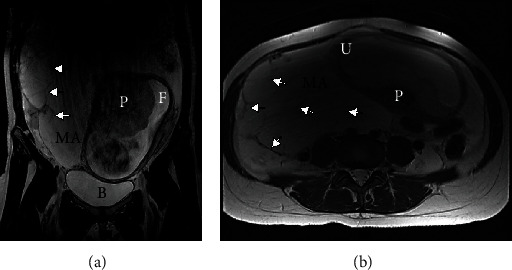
(a), (b) Abdominal and pelvic MRI without IV contrast ((a) coronal plane, (b) axial plane) shows a gravid uterus and significant amount of T2 hyperintense fluid, some of which is loculated with internal septations (arrows). B: bladder; F: fetus; IV: intravenous; MA: mucinous ascites; MRI: magnetic resonance imaging; P: placenta; U: uterus.
